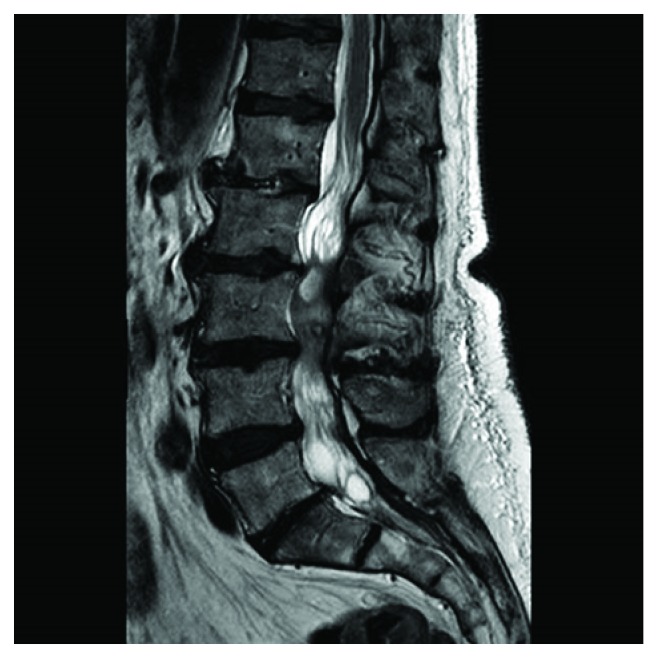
Sagittal T2-weighted MRI demonstrated evidence of degenerative changes but no involvement of the vertebral bodies or disc spaces.
